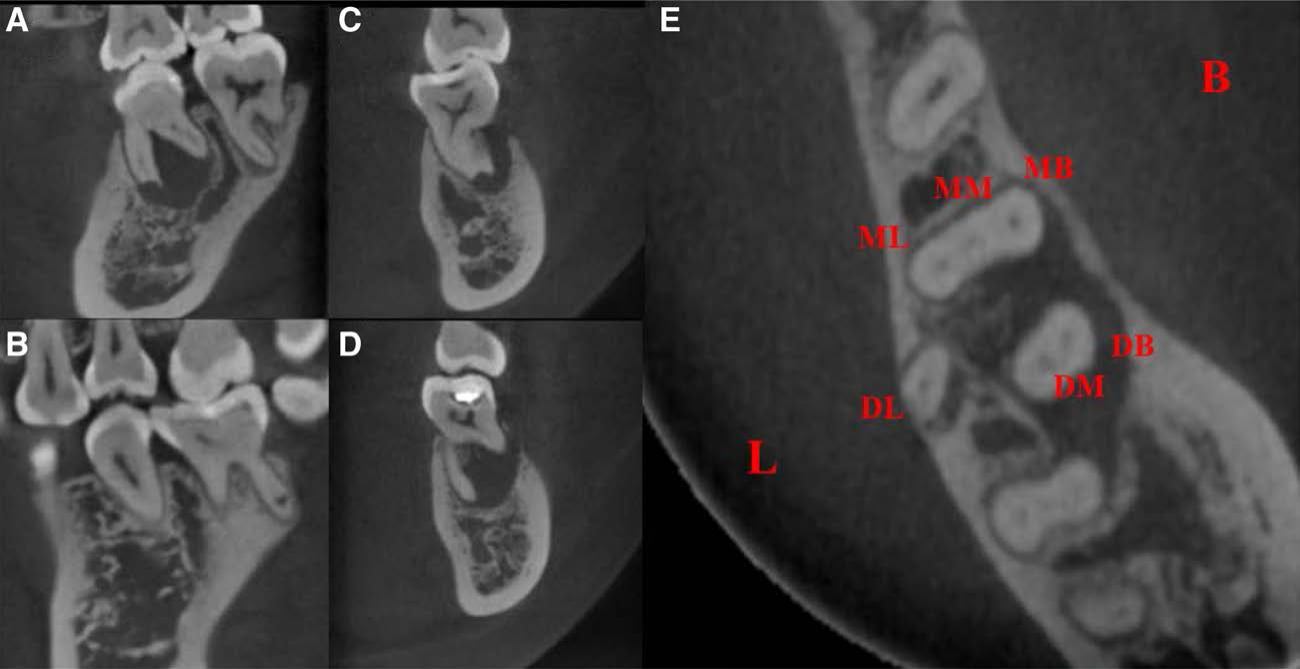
Preoperative CBCT scan of tooth 36. (A–B) Significant mesial and distal root resorption. (C–D) Apical lesion spreading to the mesial root of tooth 37 combined with alveolar bone resorption extending to the periapical area. (E) Three mesial (MB, ML and MM) and three distal (DL, DB and DM) orifices in the pulp chamber. CBCT = cone-beam computed tomography, DB = disto-buccal, DL = disto-lingual, DM = disto-middle, MB = mesio-buccal, ML = mesio-lingual, MM = mesio-middle.
